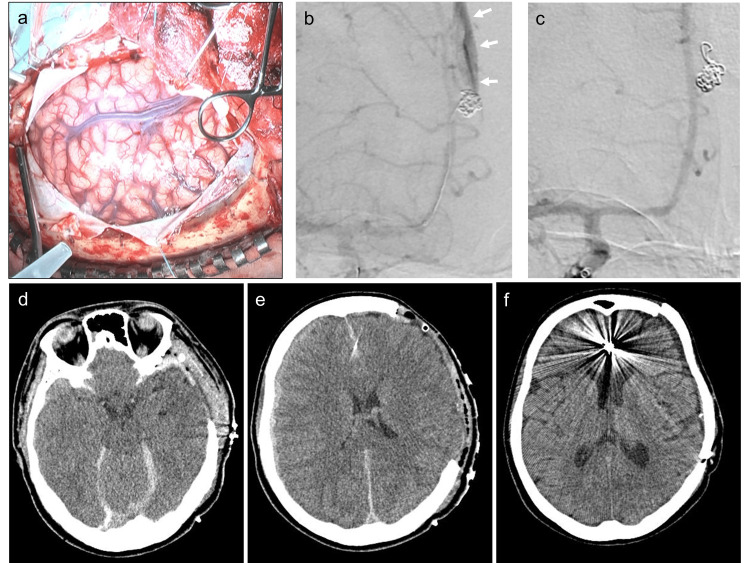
Intraoperative and postoperative imaging findings. (a) Intraoperative image showing no evidence of subarachnoid hemorrhage (SAH) or vessel injury that could cause the acute subdural hematoma (ASDH). (b) Internal carotid arteriography image showing extravasation (arrows), with the upward migration of the contrast medium along the falx. (c) Follow-up internal carotid arteriography image obtained after the completion of coil embolization showing complete aneurysm occlusion. (d, e) Computed tomography (CT) images just after coil embolization showing increased ASDH containing contrast medium around cerebellar tentorium, right convexity, and interhemispheric fissure adjacent to coil mass with no evidence of SAH. (f) CT one day after additional coil embolization showing no new lesion.
